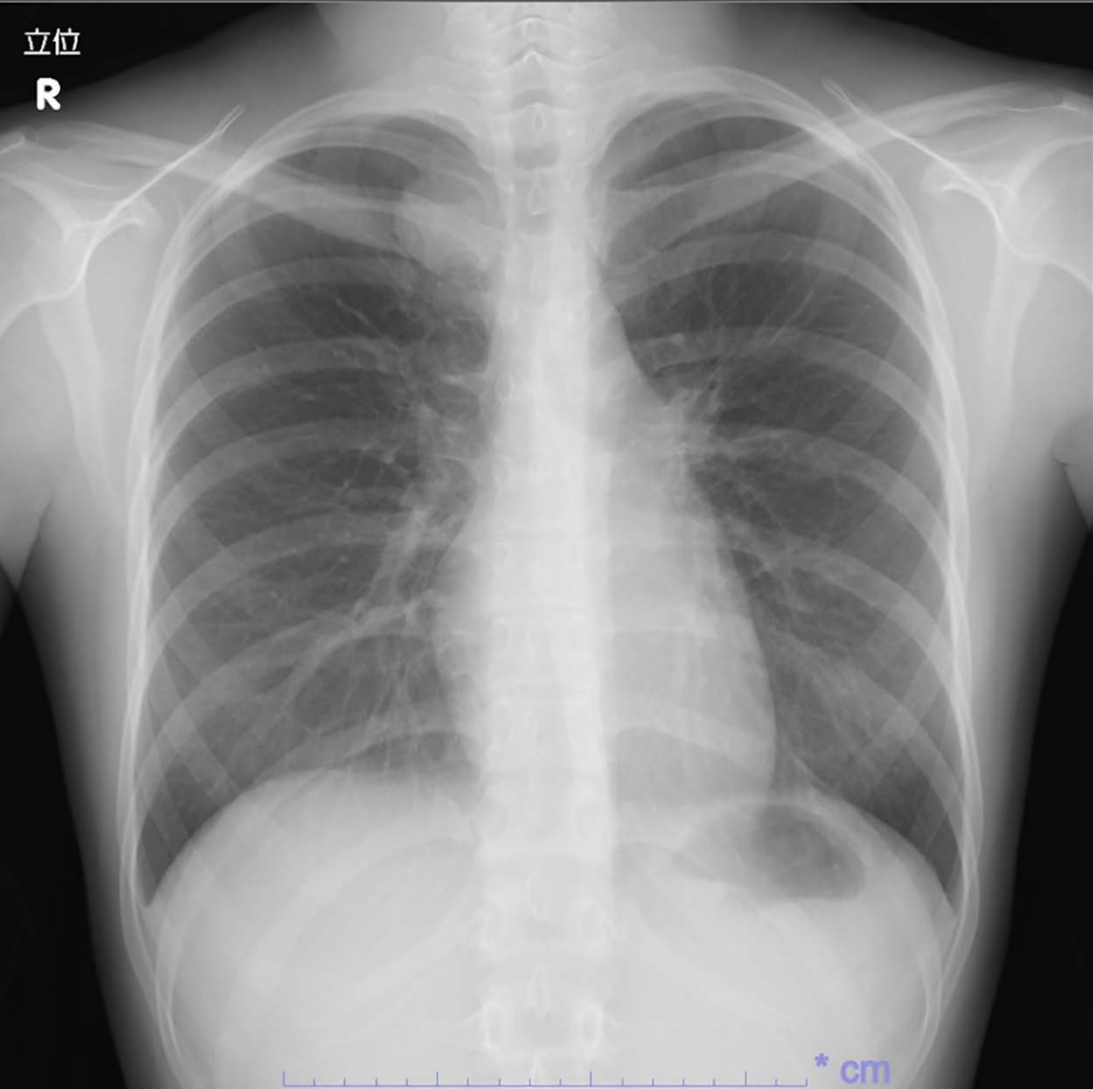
Chest radiograph. A well-defined mass of approximately 3 cm in size was observed on the mediastinal side of the right upper lung field. The silhouette sign was negative.
